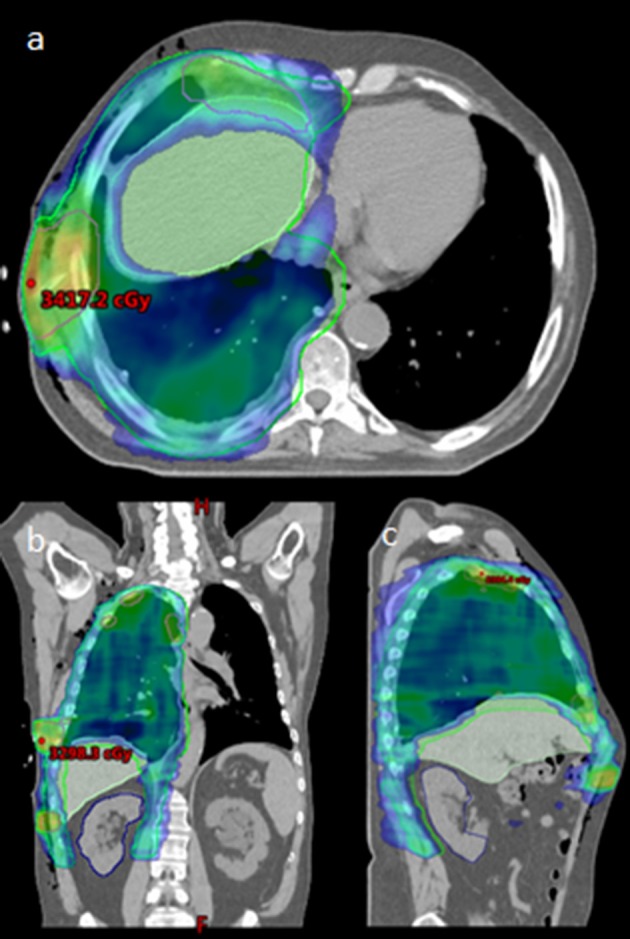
Radiation Plan for typical patient, (a): axial, (b): coronal, and (c): sagittal. Maximum point dose indicated within boosted area of PET/CT identified gross disease.
